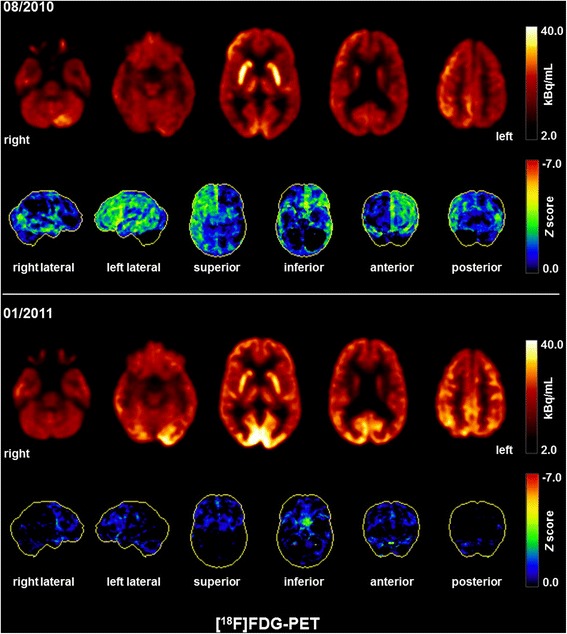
Fluorodeoxyglucose positron emission tomography (FDG-PET) findings. The initial FDG-PET scan (08/2010) depicted a global cortical hypometabolism of the left hemisphere, and, to a lesser extent, also of the right hemisphere; on the right side, hypometabolism was primarily located in the temporal lobe. Cerebellar hypometabolism was pronounced on the right side (most likely indicating crossed cerebellar diaschisis). The lower FDG-PET scan was acquired after successful immunosuppressive therapy (01/2011), indicating a nearly regular cerebral metabolism. A slight frontal hypometabolism remained most likely secondary due to frontal accentuated atrophy. Pronounced occipital metabolism is explainable by scan acquisition with open eyes. Both FDG-PET scans were performed at the Department of Nuclear Medicine of the University Hospital Freiburg after injection of 240 MBq 18FDG each (Gemini TF64 PET scanner, Philips Healthcare, The Netherlands)
